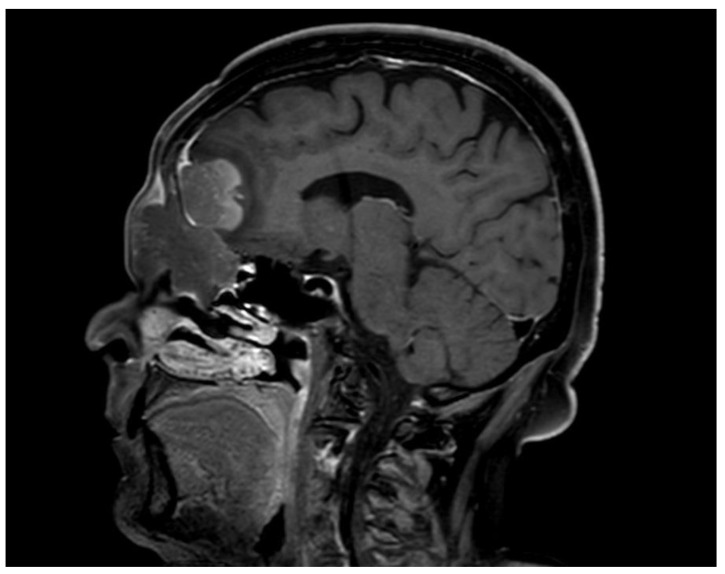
Magnetic resonance imaging (MRI) showing a partial response after chemotherapy with R-DA-EPOCH (etoposide, prednisone, vincristine, cyclophosphamide, doxorubicin, and rituximab).
